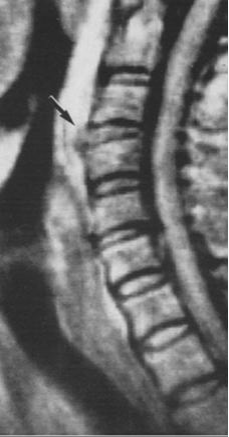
Magnetic resonance imaging was consistent with a disruption of the anterior longitudinal ligament and anterior protrusion of the intervertebral disc (black arrow).
